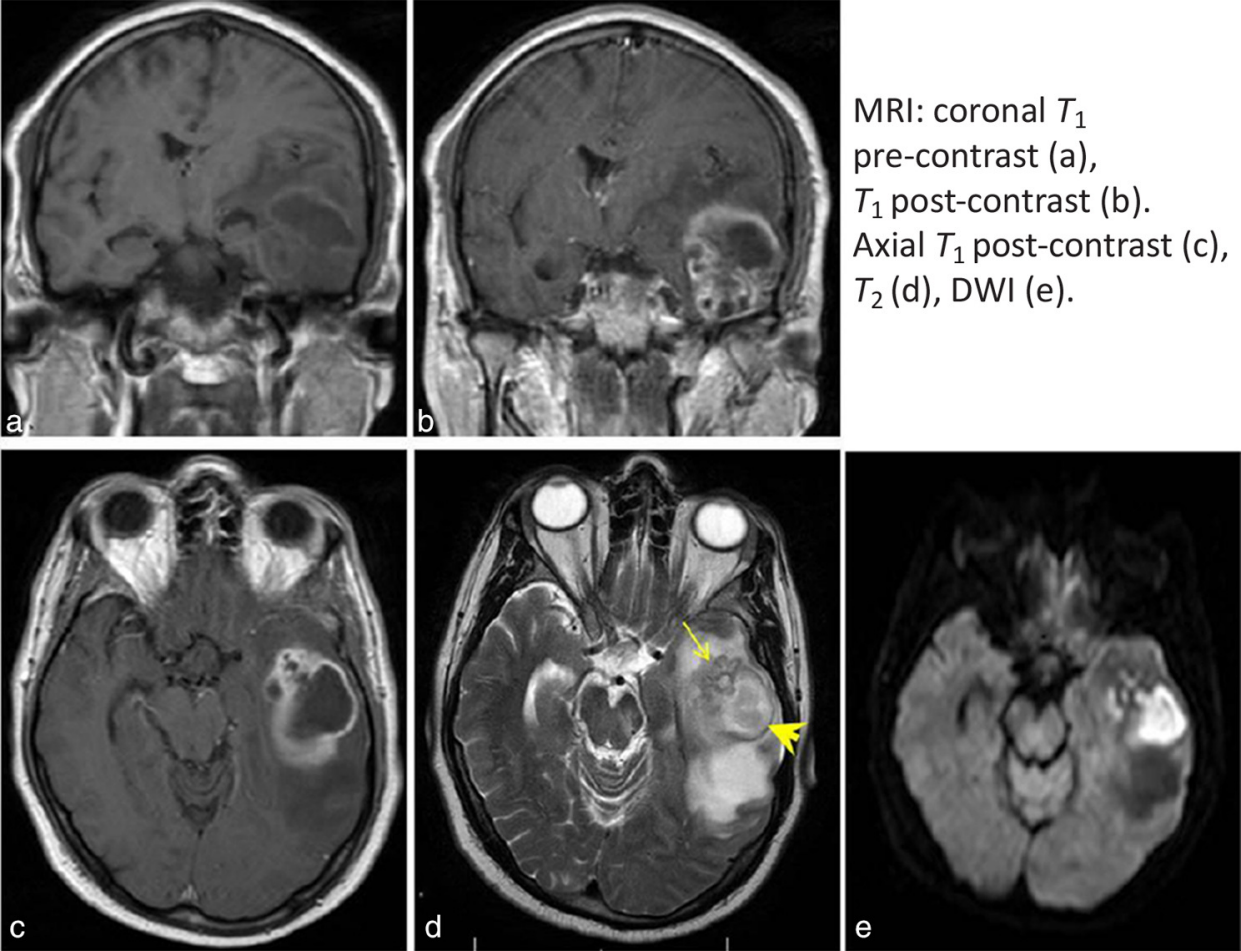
There is a large, peripherally enhancing thick-walled lesion in the left temporal lobe. The lesion comprises a larger cavity posteriorly and grape-like clustering anteriorly (arrow). The wall is *T*_2_ hypointense and *T*_1_ hyperintense (arrowhead). The contents of the lesion show restricted diffusion, which is consistent with an abscess. The diagnosis is suggested by the grape-like cluster pattern with a *T*_2_ hypointense wall, which is a characteristic feature of an actinomycotic abscess. DWI, diffusion-weighted imaging.
